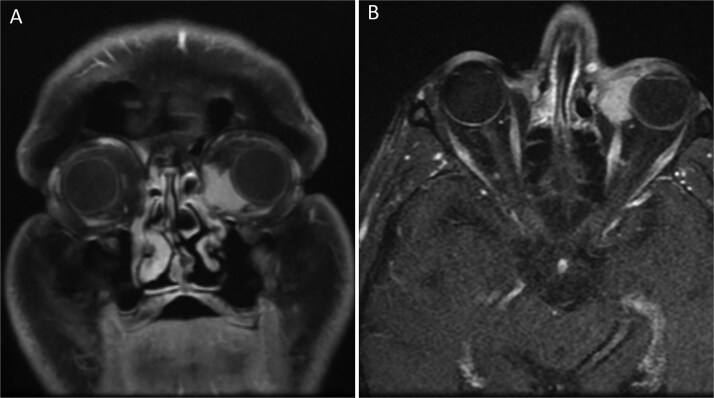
Orbital MRI images of the patient (A) Coronal T1-weighted orbital MRI showing a well-defined lesion in the left caruncular region. The mass measures ~23 × 34 mm and extends to the medial and inferior orbital walls, with homogenous contrast enhancement. No significant infiltration into adjacent structures is observed. (B) Axial T1-weighted orbital MRI showing a homogeneously enhancing mass in the left caruncular region. The lesion extends to the medial and inferior orbital walls without significant involvement of adjacent orbital structures. The mass demonstrates clear boundaries and no evidence of periorbital tissue infiltration.
